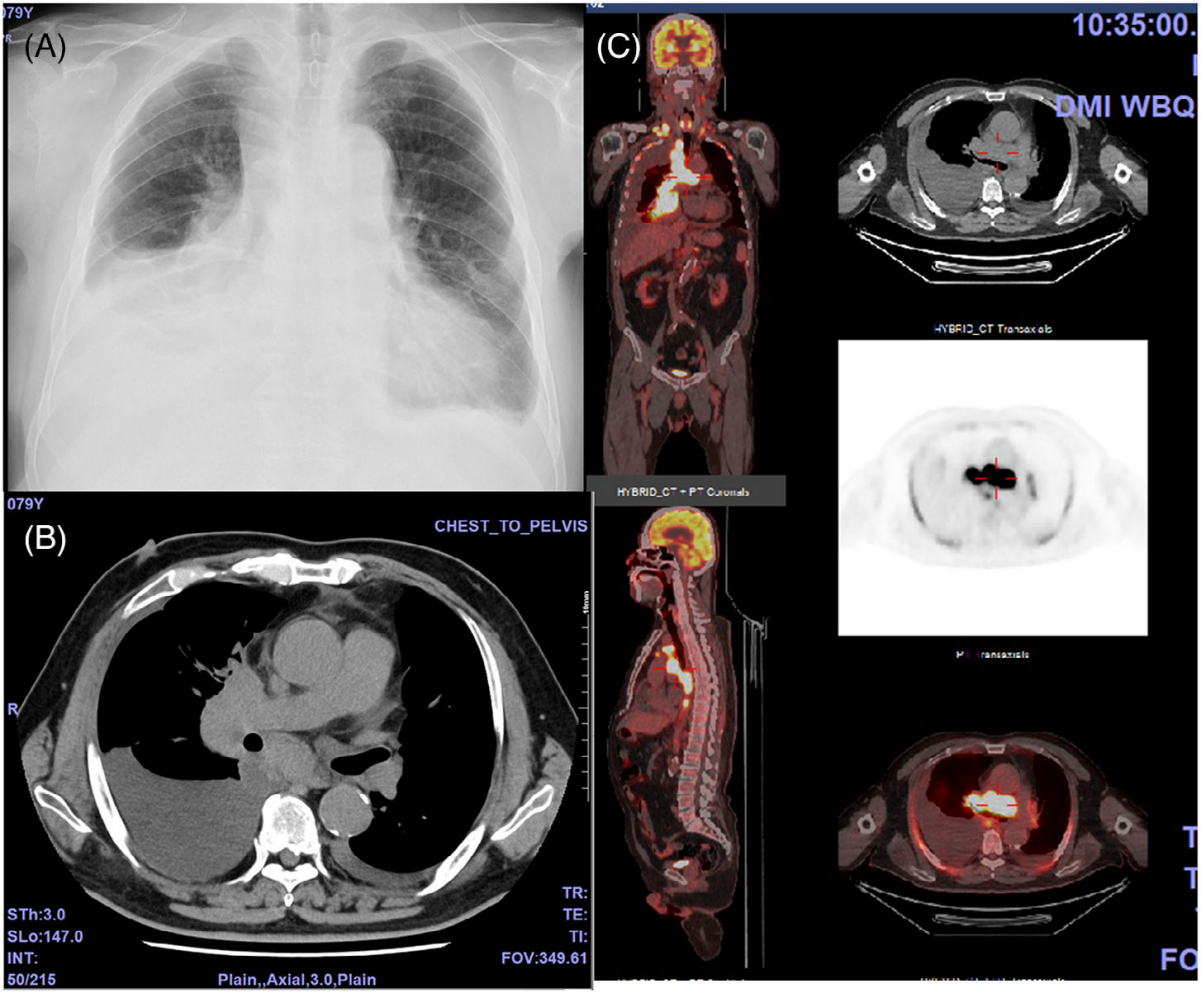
(A) Chest X‐ray indicated a large amount of pleural effusion. (B) Computed tomography revealed enlarged lymph nodes in the mediastinum and pleural effusion. (C) Positron emission tomography showed a high concentration in the mediastinal mass.
